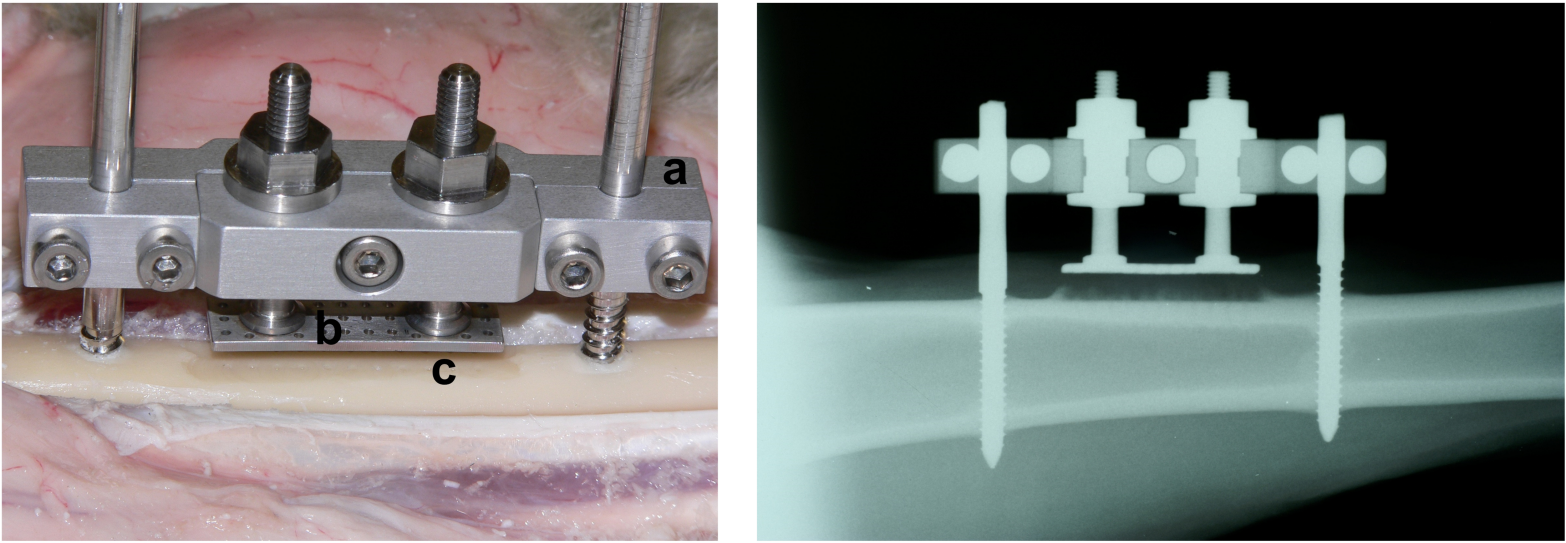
Lateral distraction study by Claes et al. [32]. Left: *In vivo* setup of the study with (a) fixator, (b) hydroxyapatite-coated titanium plate and (c) face milled medial surface of the diaphysis; right: X-ray taken 4 weeks post-op, showing traces of initial bone formation above the medial surface.
